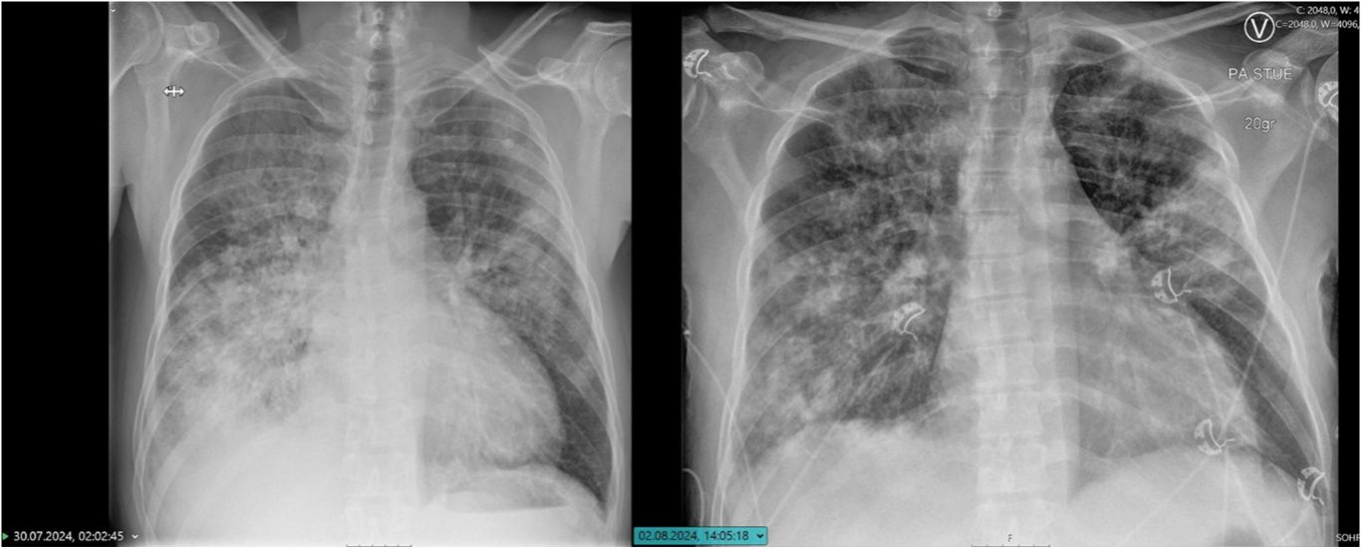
Chest X-rays from initial admission (left) and re-admission (right). The initial image shows extensive bilateral consolidations consistent with severe pneumonia and pleural effusion. On re-admission, there is persistent but slightly improved bilateral consolidation, indicating partial resolution of the pulmonary infiltrates.
